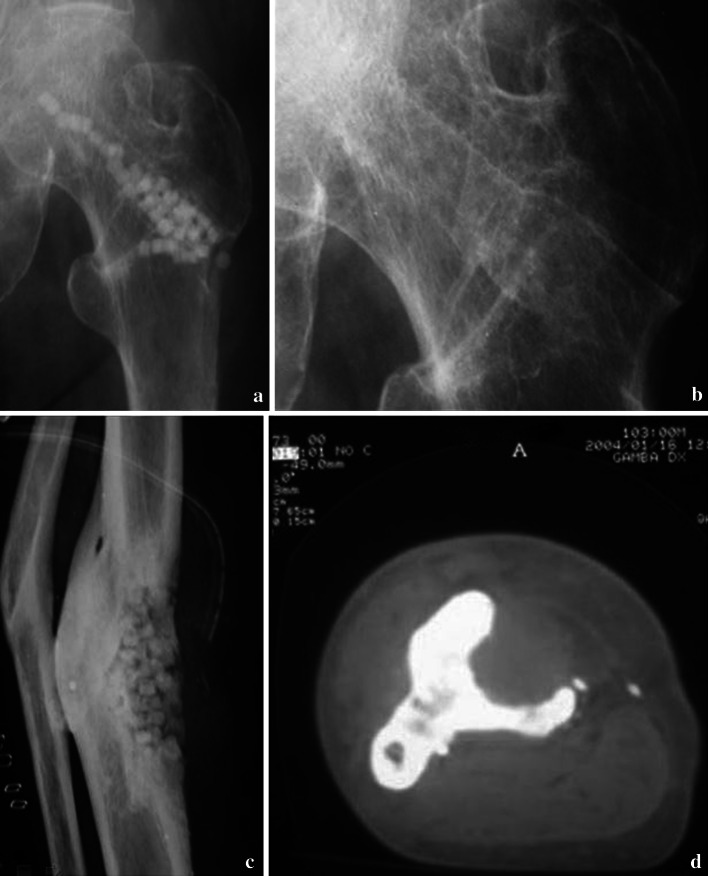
Calcium sulphate (CS): a Pellets fill the residual gap after DHS explant in a healed intertrochanteric fracture. b Two months after the CS was totally resorbed. c Antibiotic loaded CS pellets in a tibial osteomyelitis. d Three years CT scan control do not show any evidence of bone regeneration. No signs of CS were founded while the infection was healed
